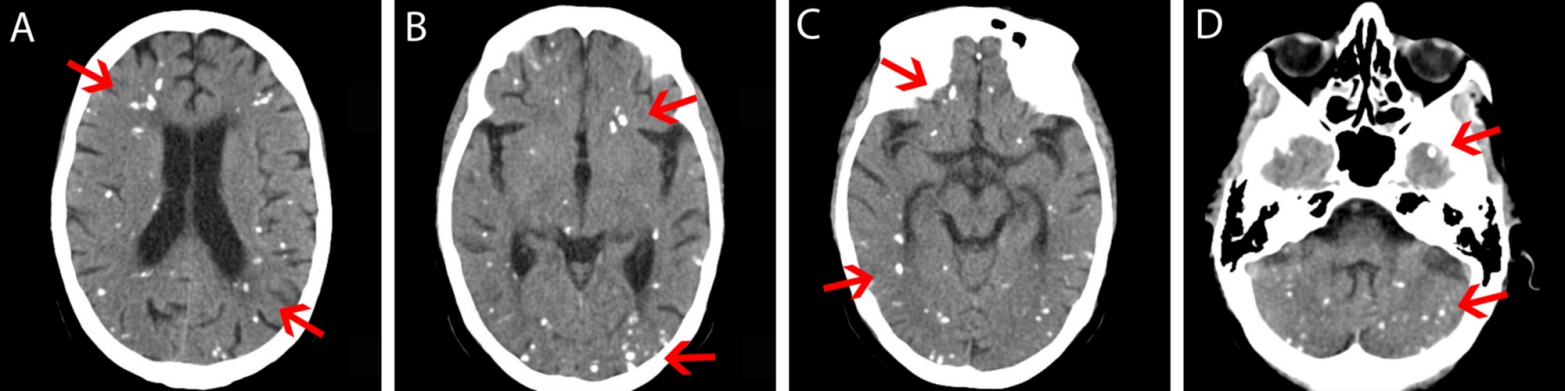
Transverse cerebral CT scan Red arrows in A-D: Micronodular lesions with calcium density distributed throughout the brain parenchyma. With gray and white substance affection. CT: computed tomography
